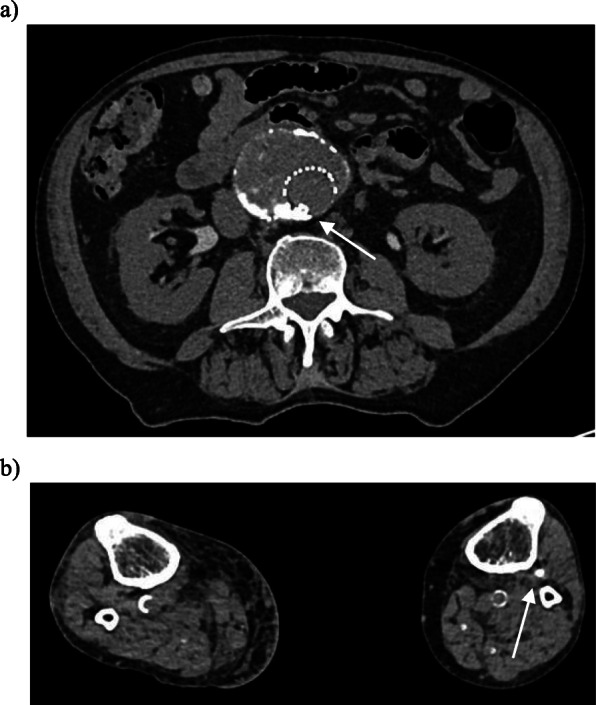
Axial non-contrast CT images of the abdomen and lower limbs performed 1 h after embolisation. Note: contrast excretion is seen from the kidneys due to contrast administered at the time of the angiography procedure. a Non-target embolisation of glue is seen (white arrow) in the stent graft lumen (arrow). b Axial non-contrast CT at the level of the calf demonstrates non-target embolization within the left anterior tibial artery
